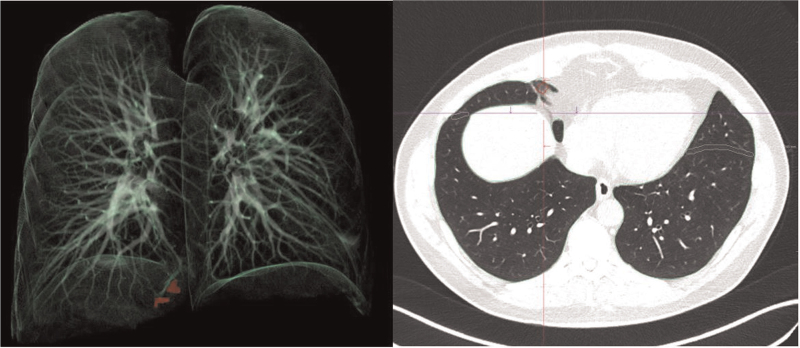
3D reconstruction and axial slices of the lungs (*syngo*.via CT Pneumonia Analysis prototype). Analysis of a 58-year-old male patient who had undergone chest CT as preoperative measurement (for hip replacement) to reduce infection risk in the pandemic setting. This patient was rt-PCR-negative for SARS-CoV-2.
